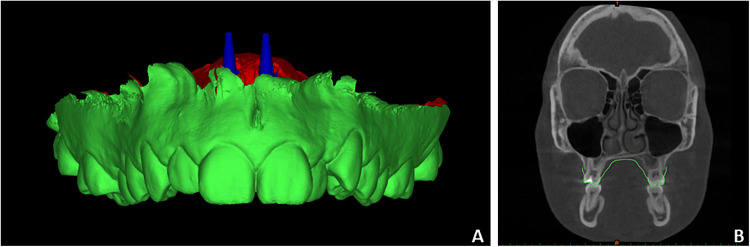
Example of single.stl file generated merging IOS and equivalent miniscrews (A) and imported into mimics software with CBCT scans (B).
